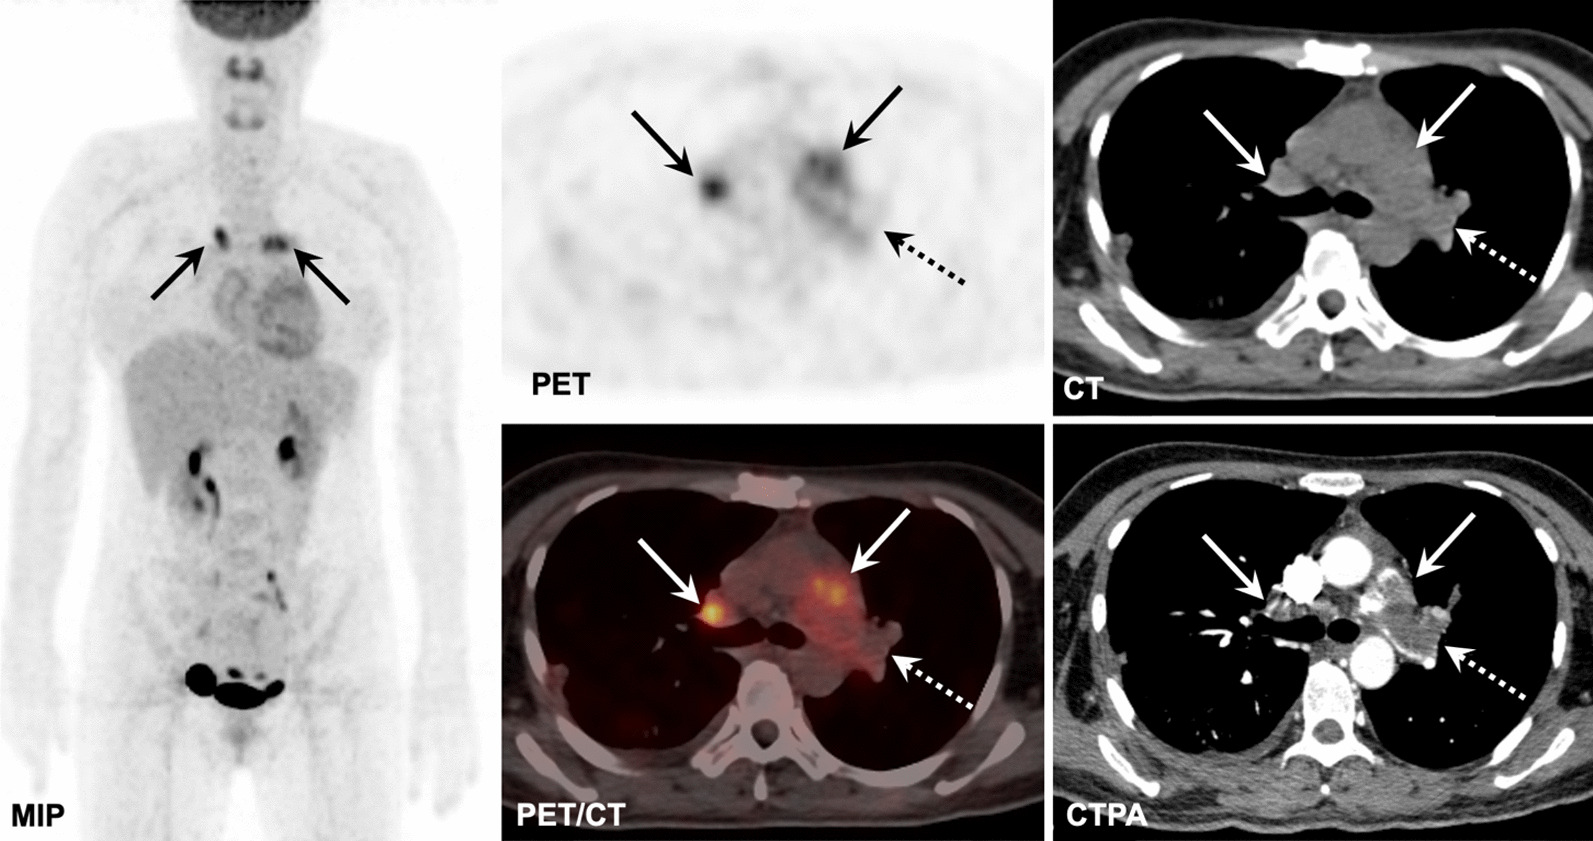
A 44-year-old woman detected with the coexistence of PAS involving PT, RPA, and LPA with SUVmax of 6.2 (solid arrows), and PTE involving LPA with SUVmax of 1.6 (dotted arrows) on FDG PET/CT and CTPA images
